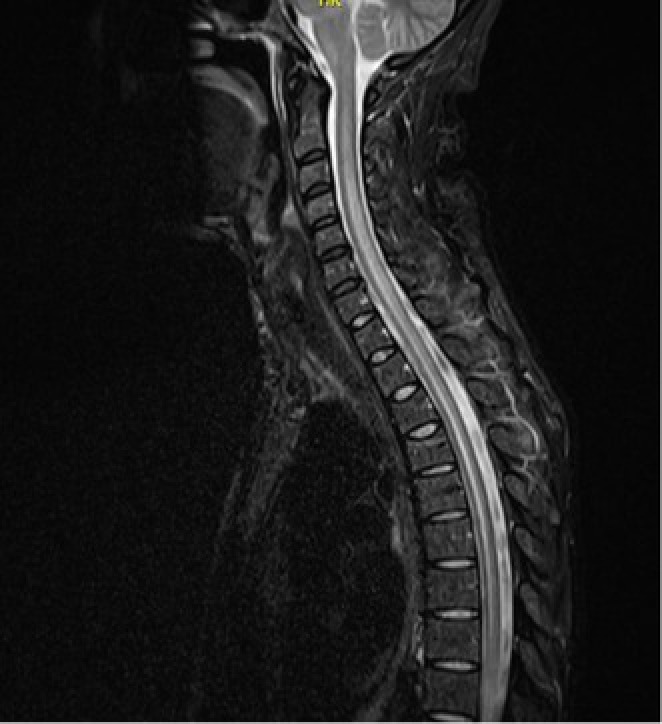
FIGURE 2 Acute inflammatory myelopathy: Sagittal T2‐weighted fat‐suppressed MRI spinal magnetic resonance imaging demonstrates a longitudinally extensive intramedullary hyperintense signal involving the cervical and thoracic spinal cord, associated with mild cord.
